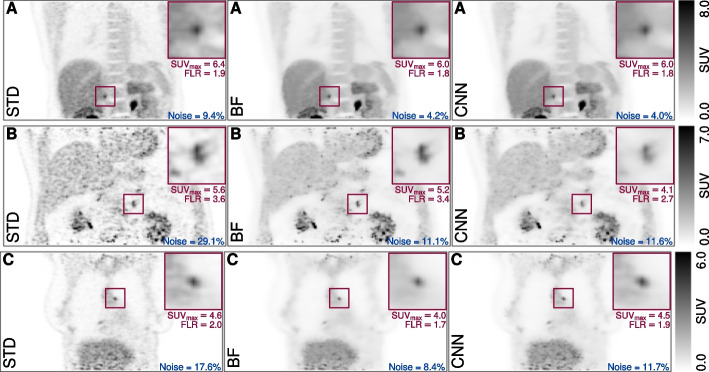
Examples of the differently processed data. Left: vendor-provided/unprocessed; middle: BF processed; right: CNN processed. The top row A shows an example ($$[^{18}\text {F}]$$L-DOPA) of nearly identical performance of BF and CNN. The middle row B shows an example ($$[^{18}\text {F}]$$FDG) with notable reduction of $$\text {SUV}_\text {max}$$ after CNN processing, which is not present after BF processing. The bottom row C shows an example ($$[^{18}\text {F}]$$FDG) where CNN performs notably better then BF regarding $$\text {SUV}_\text {max}$$
